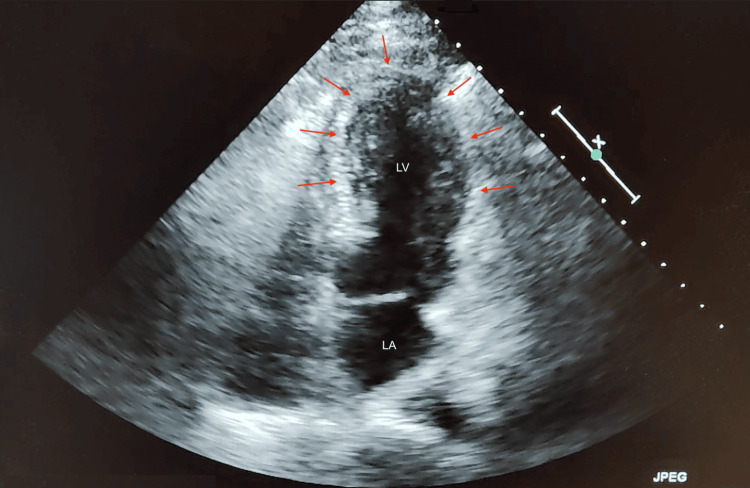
Echocardiogram without contrast medium in apical two-chamber view. Left ventricular (LV) systole showing hypokinesis of the mid and apical segments (red arrows).
